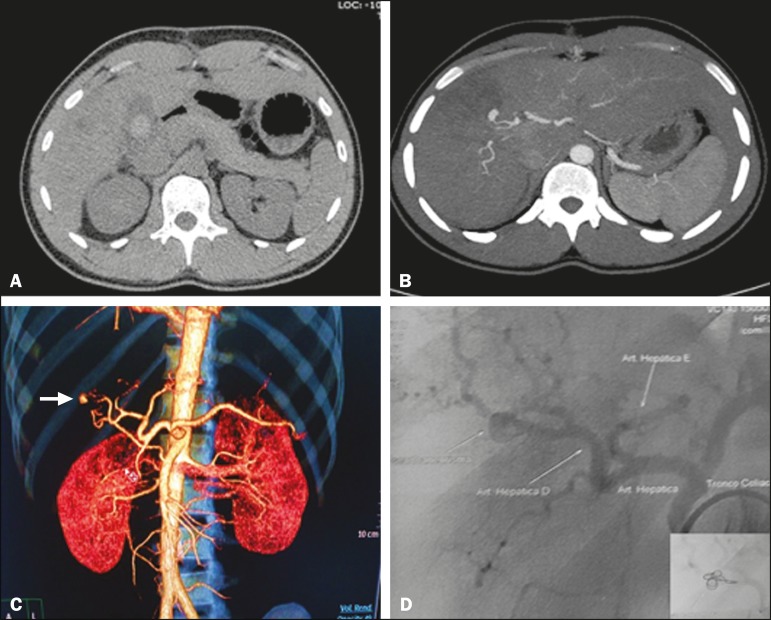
A: Noncontrast axial CT showing spontaneously hyperdense
